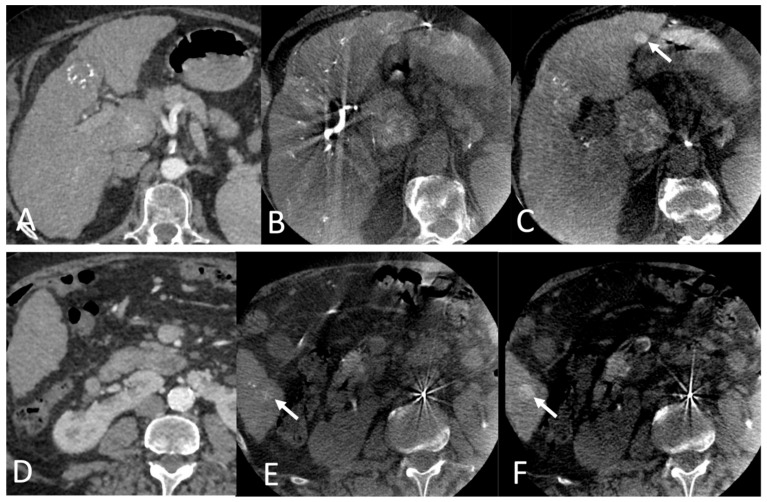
(A,D): CT axial images at the late arterial phase. (B,C,E,F): CBCT axial images at the arterial (B,E) and parenchymal (C,F) phases: Hypervascular incidentaloma (white arrows) detected in segments III and VI by per-interventional CBCT during a TACE performed one month after the CT scan. Note the better visibility of hypervascular nodules in the parenchymal phase of dual-phase CBCT.
